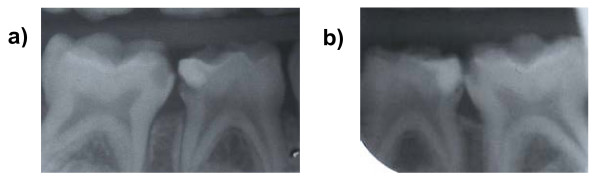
Radiographs of two matched carious lesions in tooth 85 (LRE) – radiograph a) and tooth 75 (LLE) – radiograph b). Patient randomisation number 92.
